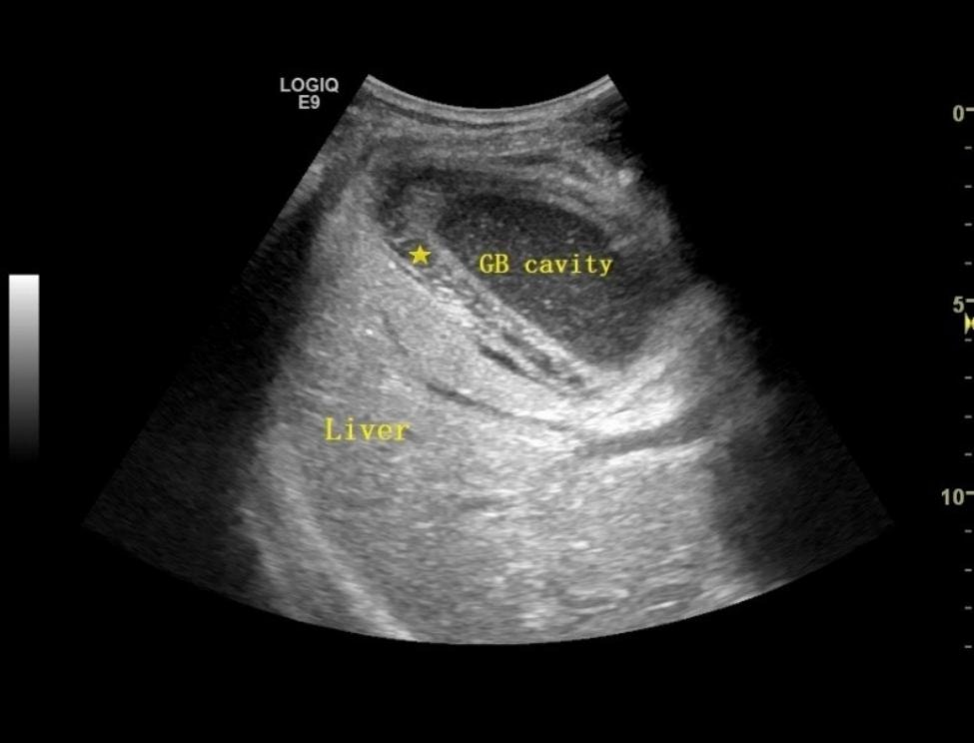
Ultrasound image of acute cholecystitis demonstrating thickened gallbladder wall (star) and dotted echogenicity in the gallbladder cavity. GB, gallbladder
